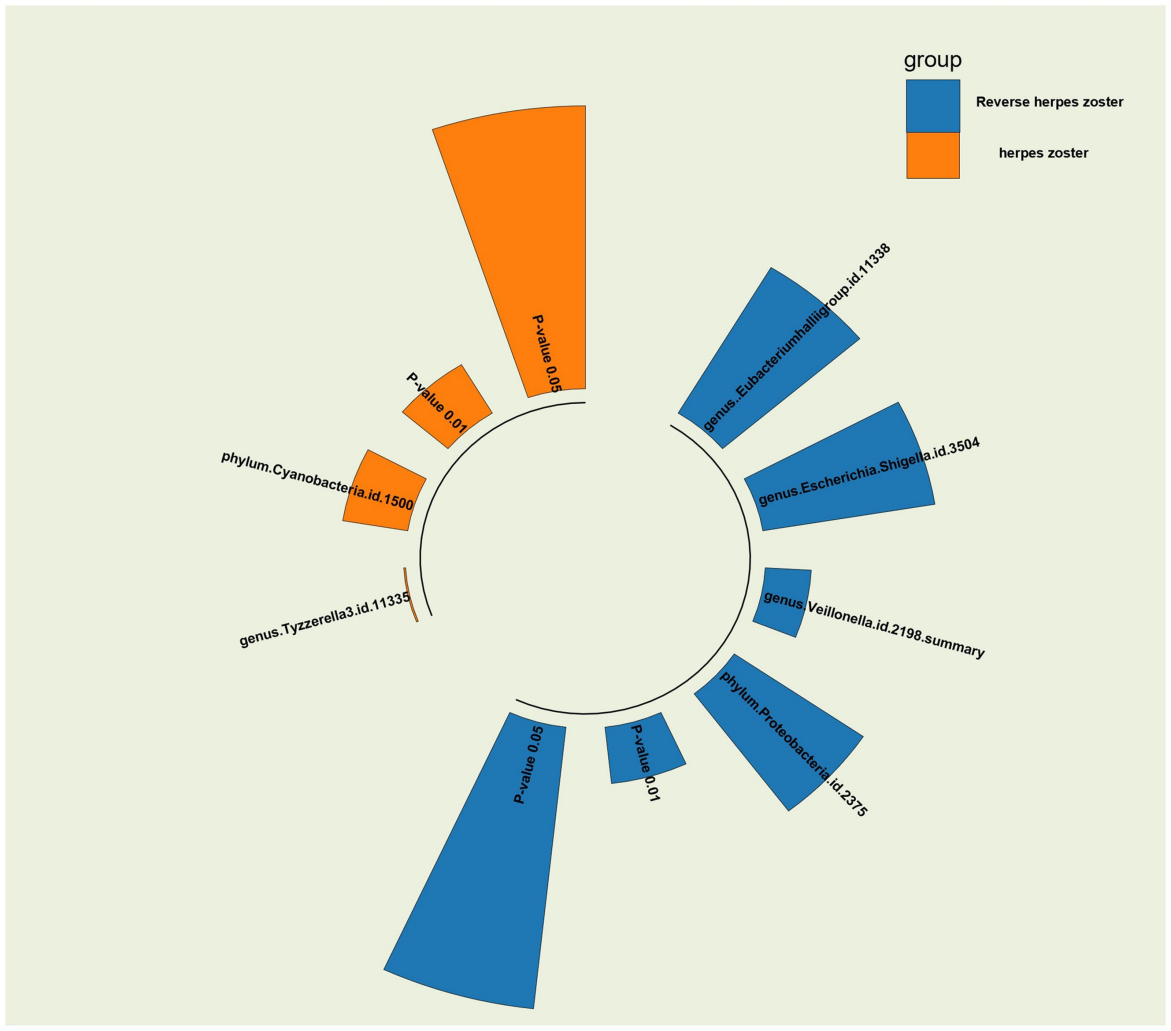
p*-value radiographs of the results in MR and inverse MR analyses of the gut microbiome and herpes zoster for the IVW method are shown and visualized against *p*-value = 0.05 versus 0.01.
